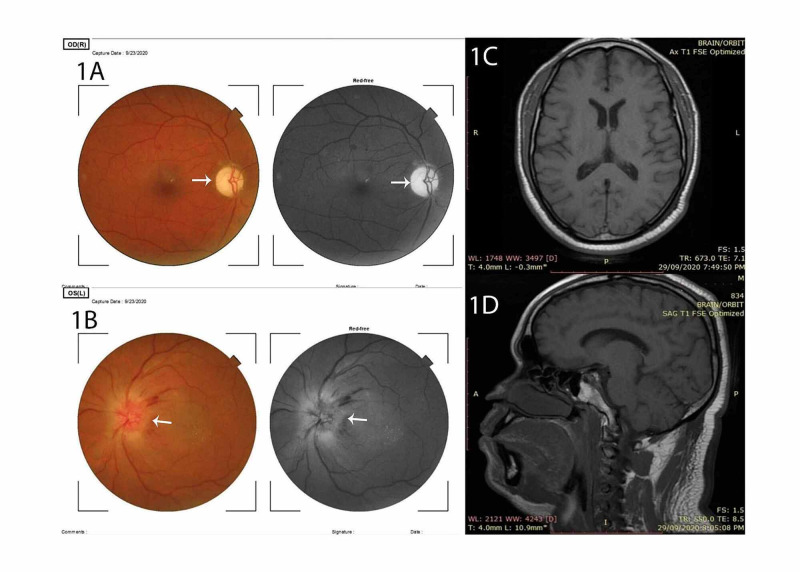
Pane 1A shows the fundus photographs of the right eye while 1B represents the left eye. Panes 1C and 1D show the MRI of the brain. The optic disc of the right eye is markedly pale while that of the left is markedly edematous and elevated, indicated by the white arrows. There is no intracranial mass in the MRI of the brain and orbit.
